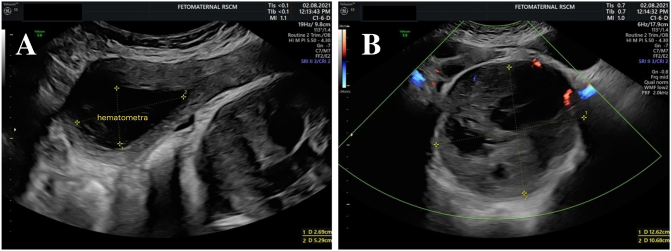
Abdominal ultrasound of the patient showing (A) hypoechoic mass sized 269 × 529 mm in the uterine cavity suspected for hematometra and (B) homogeneous solid mass sized 126 × 106 mm on the left adnexa suspected for myoma with cystic degeneration.
